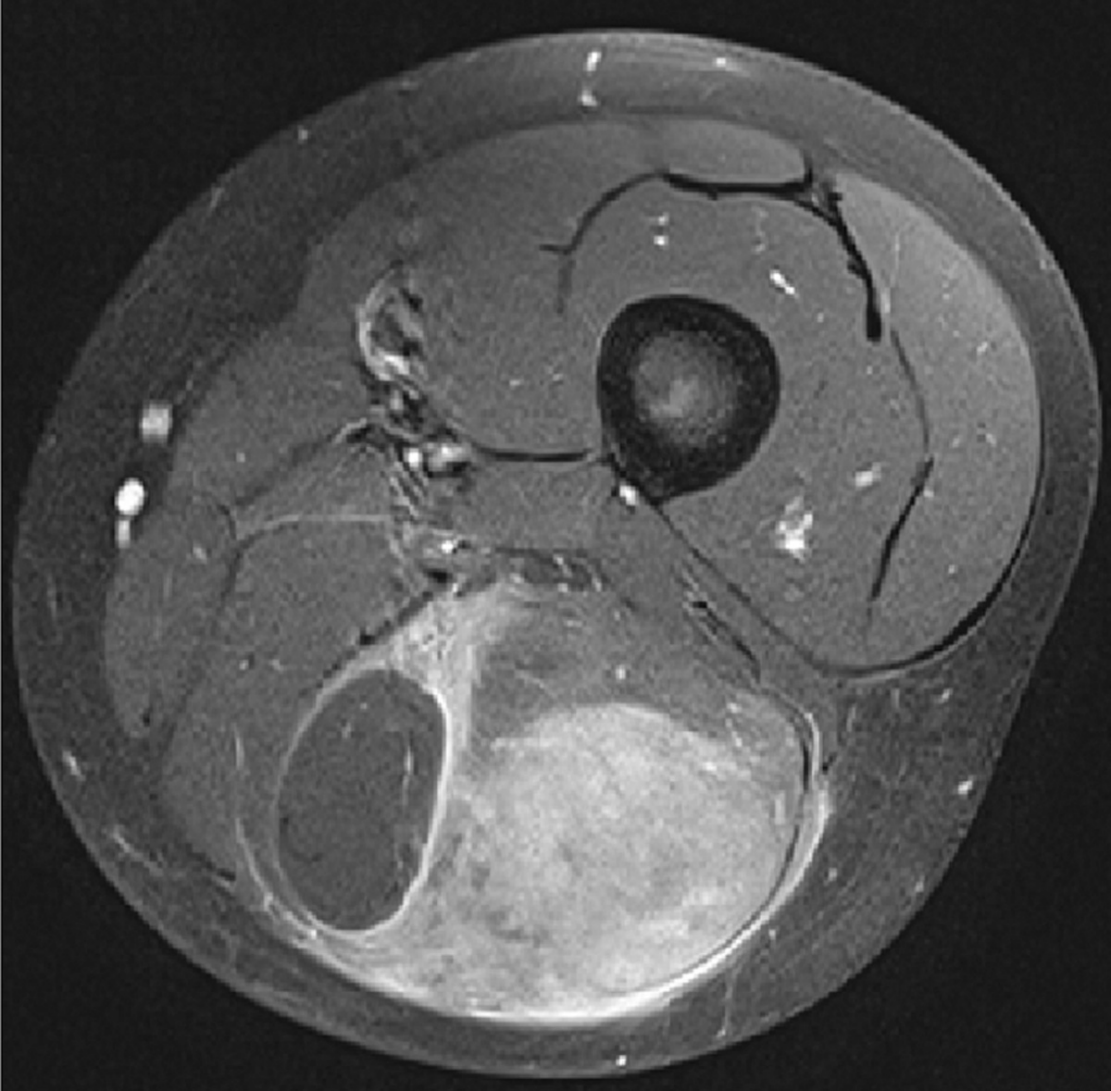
Axial MRI of the left thigh delineates an ill-defining tumor mass within the long head of the biceps femoris muscle with vivid uptake of contrast media while measuring 4 × 3 × 9 cm in size.
